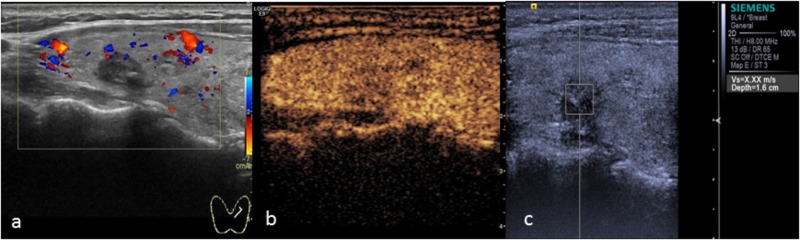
Thyroid adenoma. (a) Color Doppler ultrasound showing circumferential blood flow around the nodule. (b) CEUS showing ring enhancement. (c) The SWV value was 2.13 m/s.
